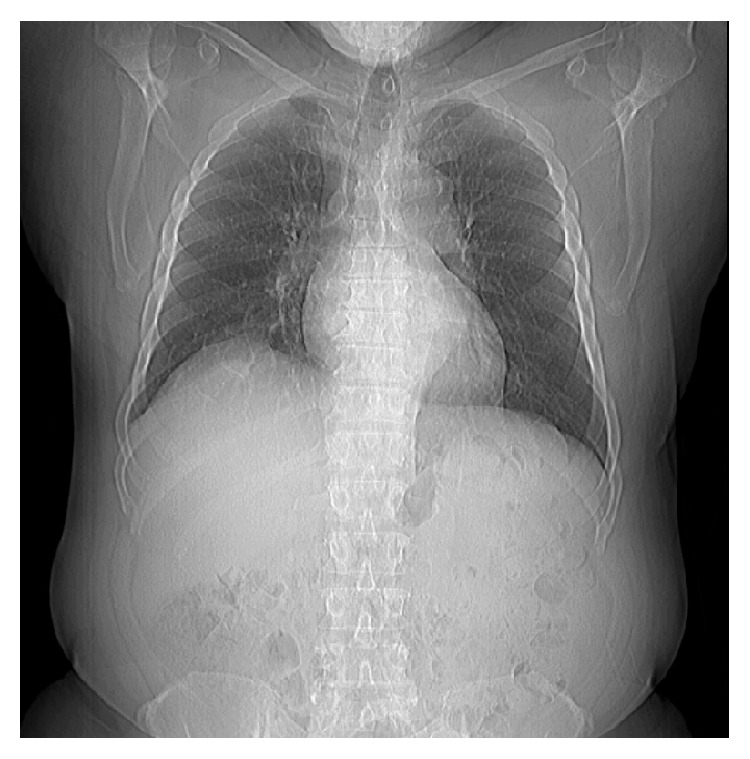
Admission CT scan did not show pulmonary infiltration.
